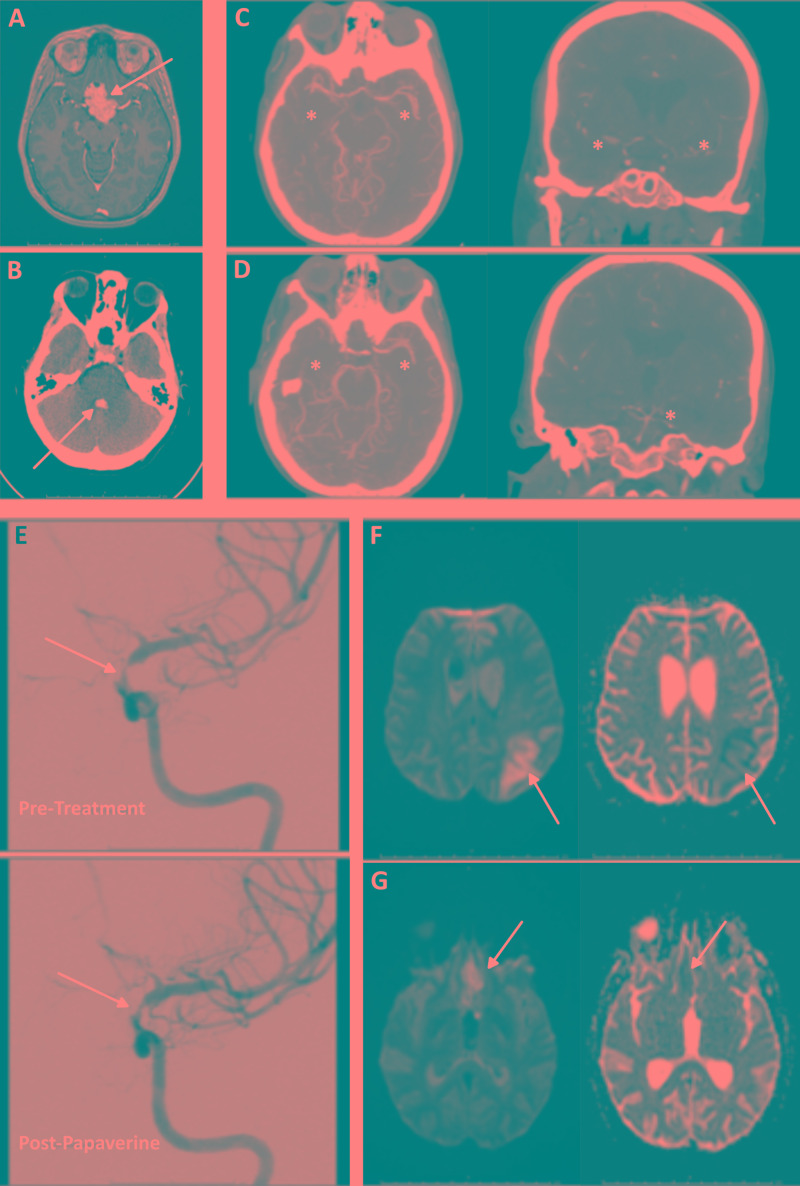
Case 1: 36-year-old woman Preoperative contrast-enhanced T1-weighted MRI reveals a large suprasellar mass with prepontine cisternal extension, encasement of the left proximal PCA, and partial encasement of bilateral supraclinoid ICA and A1 segments (arrow). (B) CT of the head shows IVH within the fourth ventricle on POD 0 (arrow). (C) CTA of the head on POD 16 suggests delayed cerebral vasospasm in the ACA and MCA territories (asterisks), worse on the left than the right side. (D) Vasospasm is also apparent in the PCA territory (asterisks), worse on the left side. (E) Digital subtraction angiography reveals severe vasospasm in the left paraclinoid ICA (arrow). (F) Left PCA infarct (DWI, then ADC) (arrows). (G) Bilateral ACA infarcts (DWI, then ADC) (arrows) CTA, CT angiography; PCA, posterior cerebral artery; ICA, internal carotid artery; IVH, intraventricular haemorrhage; ACA, anterior cerebral artery; MCA, middle cerebral artery; DWI, diffusion-weighted imaging; ADC, apparent diffusion coefficient; POD; postoperative day
